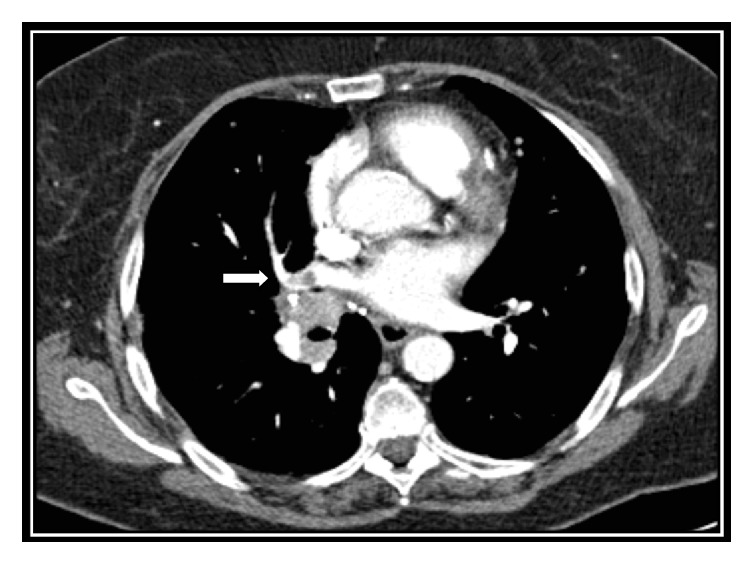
PET-FDG computed tomography scan performed 6 months after surgery and radioiodone ablation. The right superior pulmonary vein appears occluded by a thrombus (arrow).
